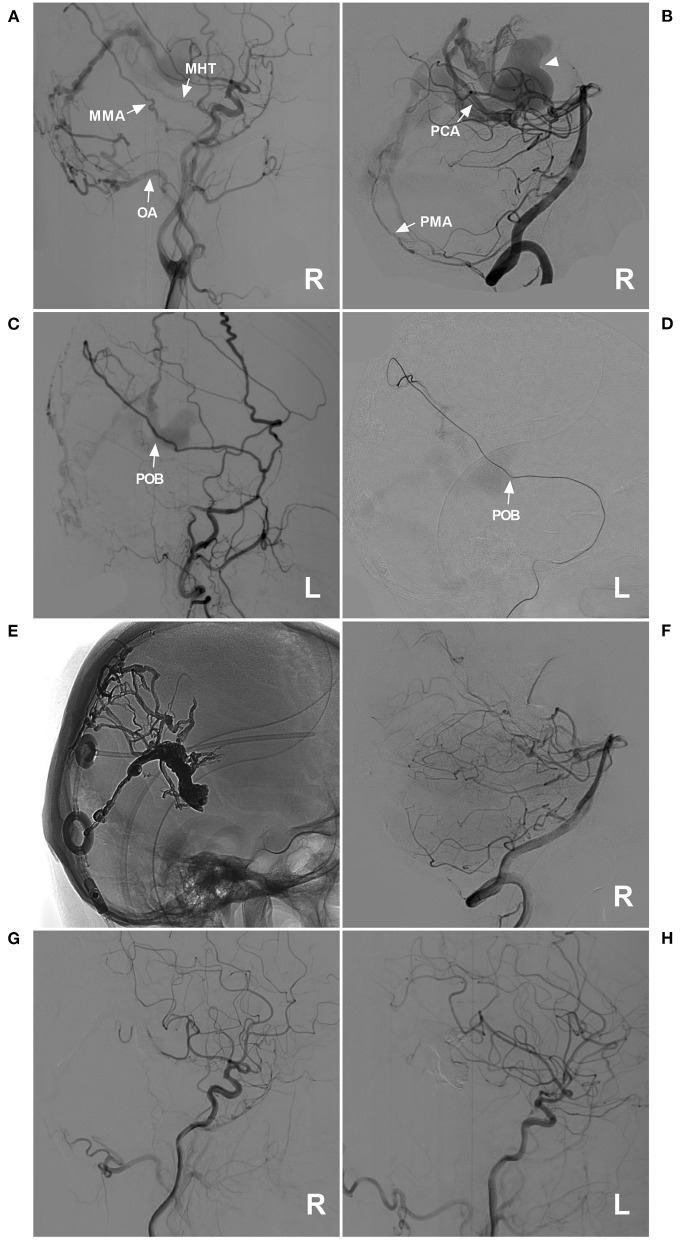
Successful type IIa EVT for a DAVF of the posterior falx cerebri. (A) Lateral view DSA of the right carotid artery showed a DAVF at the posterior falx cerebri that was supplied by the MHT, the POB of the MMA, and the OA (arrows). (B) DSA of the right VA showed that the dural branch of the PCA (arrow) and the PMA (arrow) supplied the DAVF. The dilated draining vein is indicated by a triangle. (C,D): Lateral view DSA of the left ECA (C) and superselective angiography of the POB (D) showed that the POB was normally developed and straight (arrows). (E) X-ray film showing the Onyx casting and previously established ventriculoperitoneal shunt. (F,H) Images of the right VA (F), right carotid artery (G), and left carotid artery (H) showed that the EVT was complete. DAVF, dural arteriovenous fistula; DSA, digital subtraction angiography; ECA, external carotid artery; EVT, endovascular treatment; L, left; MHT, meningohypophyseal trunk; MMA, middle meningeal artery; OA, occipital artery; PCA, posterior cerebral artery; PMA, posterior meningeal artery; POB, parieto-occipital branch; R, right; VA, vertebral artery.
